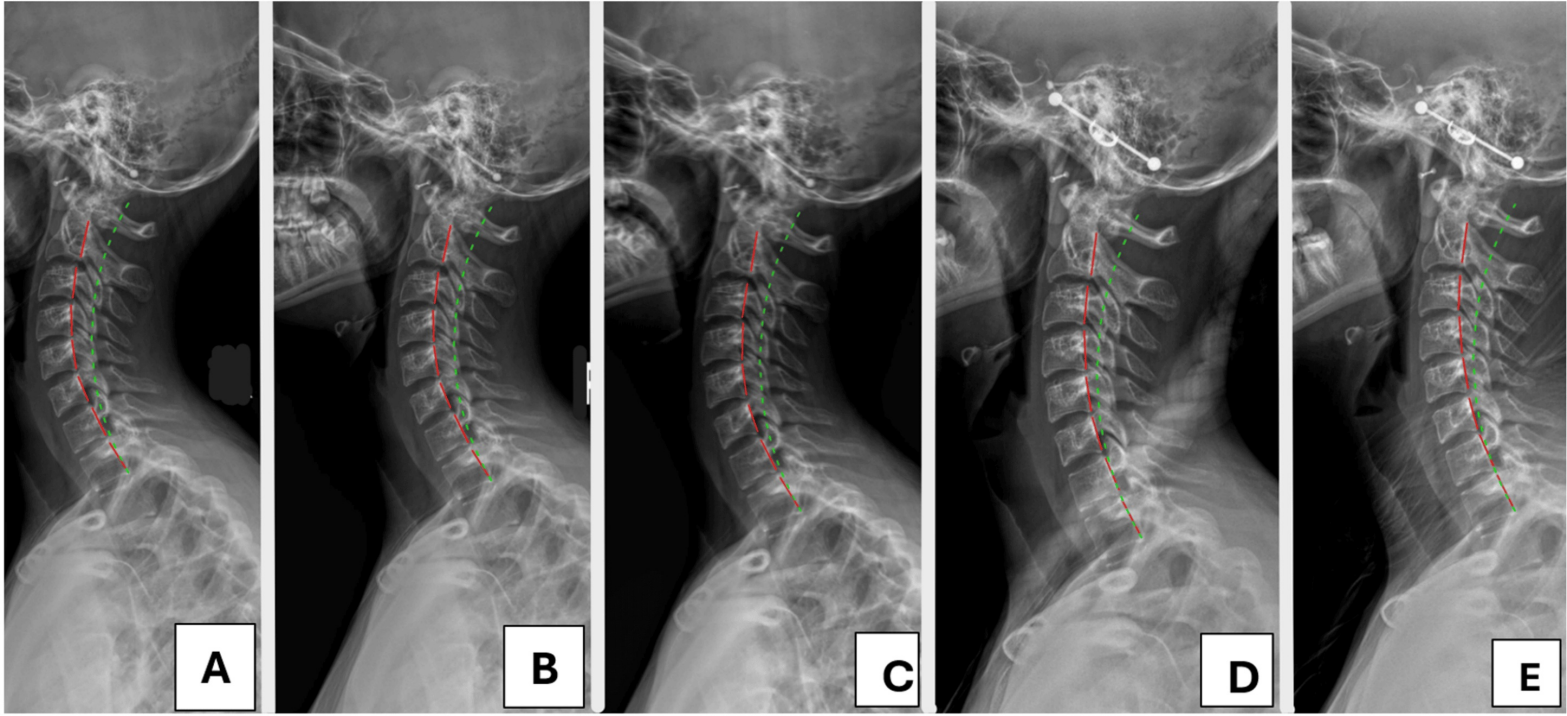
Lateral cervical radiographs from the initial (A) to the final follow-up examination (E). The green line represents the normal cervical lordosis, and the dashed red line represents the patient’s posterior vertebral lordosis contour. The patient progressed from significant hyperlordosis in panel A to a near normal lordosis following treatment and demonstrated stability with long-term follow-up in panel F.
